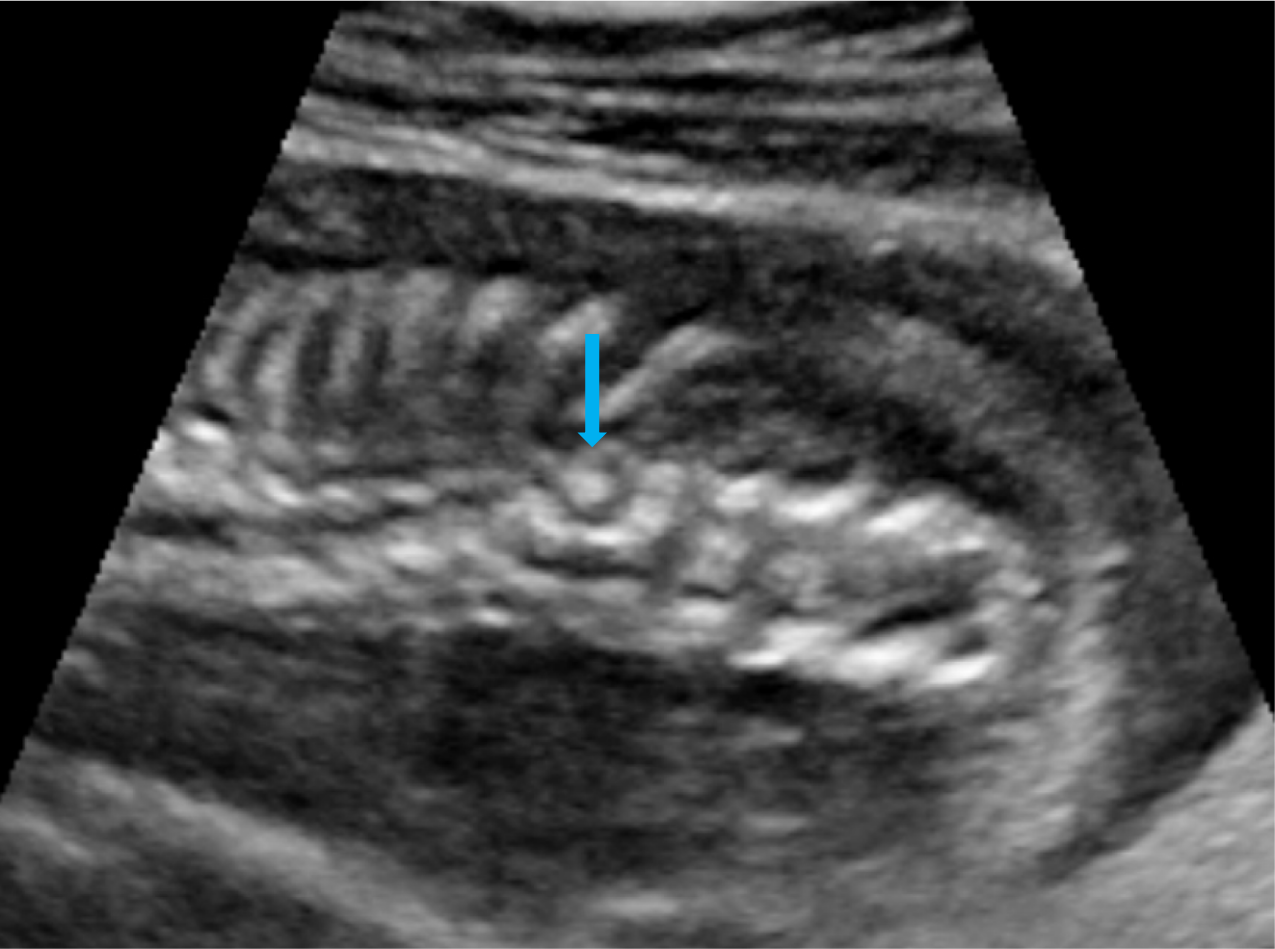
Ultrasound image of fetal spine in the coronal plane showing wedge‐shaped vertebra (blue arrow).
